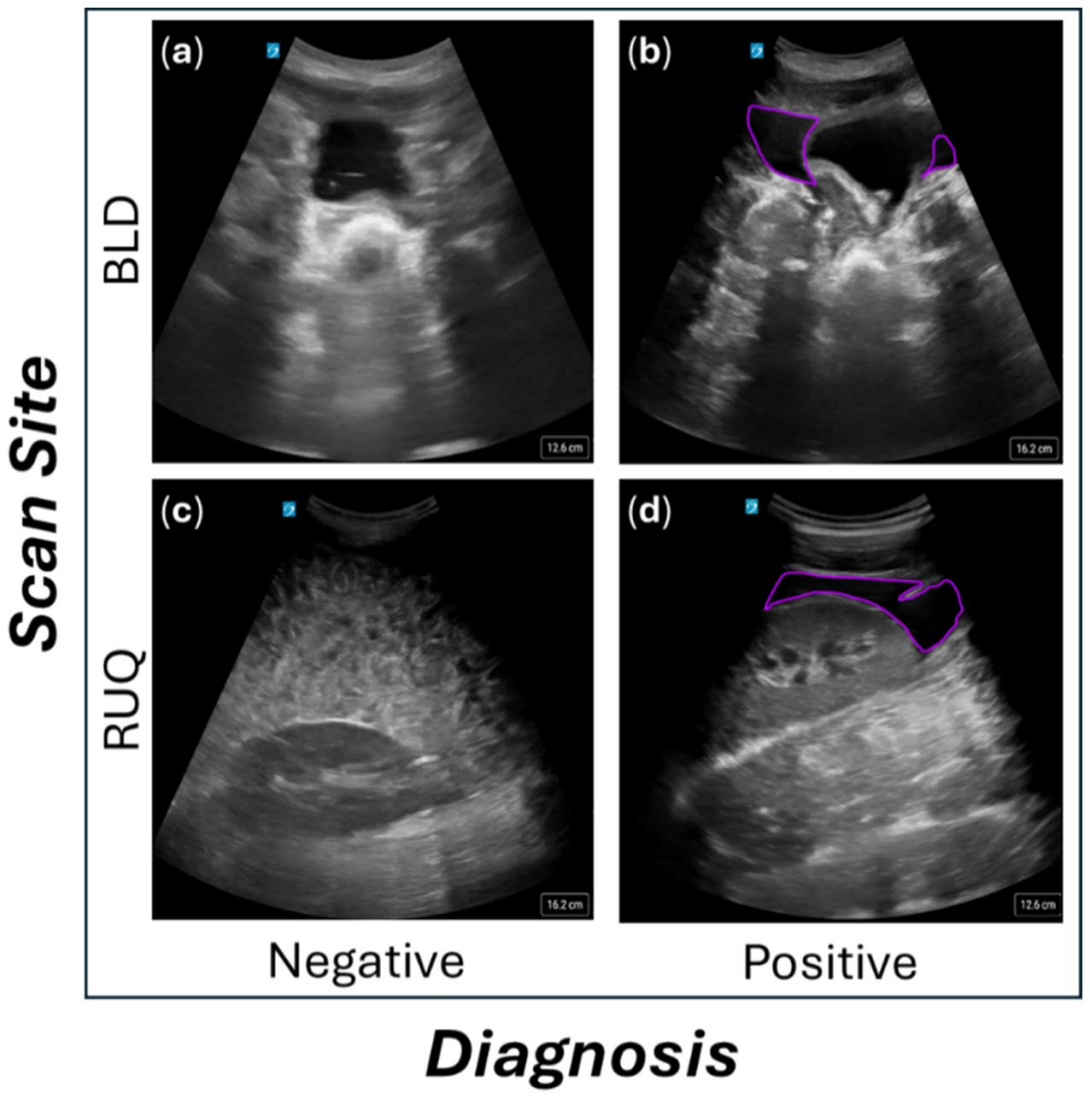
Representative ultrasound scan frames from the abdominal scan sites. Images on the top row are from the BLD scan site for negative (a) and positive (b) injury classifications. Images on the bottom row are from the RUQ scan site for negative (c) and positive (d) injury classifications. US images with injury were annotated with a purple perimeter around the fluid, located around the left and right side of the bladder for BLD and above the kidney for RUQ.
